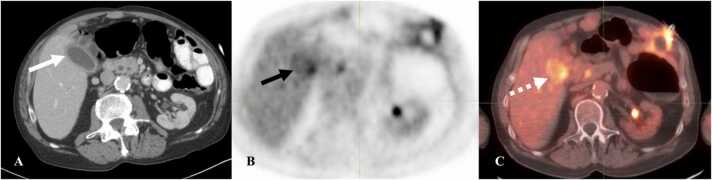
Immune-mediated cholecystitis in an 88-year-old woman with squamous cell cancer of the left tonsil on pembrolizumab. A. Contrast-enhanced axial CT abdomen image shows distended gallbladder with enhancing mucosa and pericholecystic fluid (white arrow) suspicious for cholecystitis. B. Axial PET/CT image shows uptake in the gallbladder wall (black arrow). C. Fused axial PET/CT image shows FDG uptake in the gallbladder wall suggesting cholecystitis (dashed white arrow).
